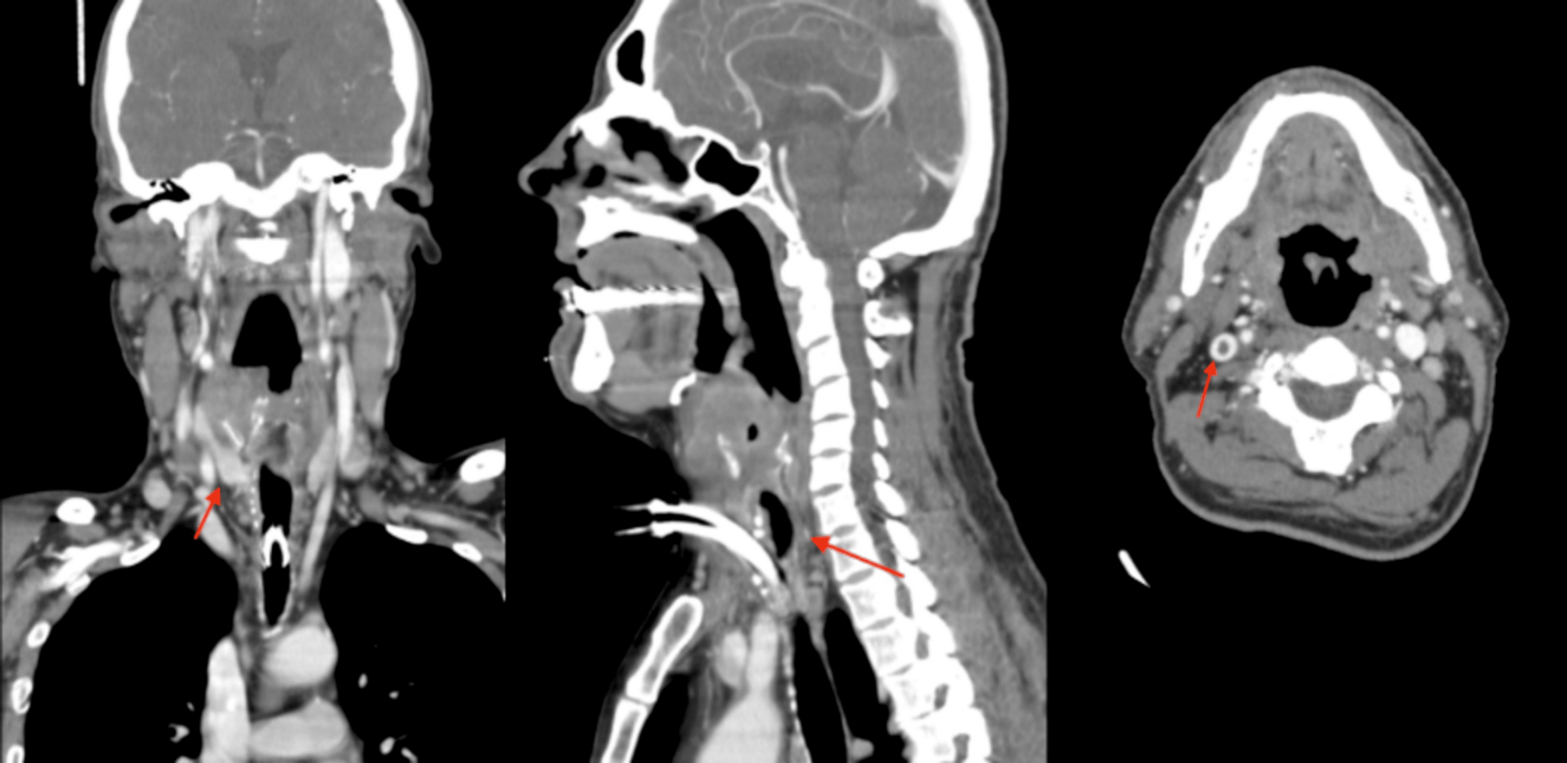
CT scan of the head and neck (multiple views) CT scans of the head and neck show bulky laryngeal mass with transglottic extension and invasion of the thyroid cartilage [red line, left two images], as well as thrombosis of the internal jugular vein (red line, right image).
